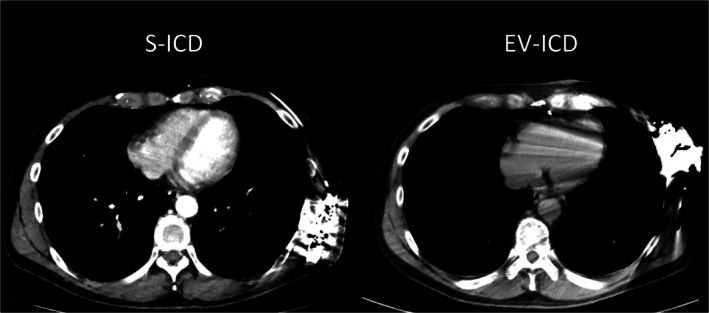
Pre‐ and postconversion computed tomography demonstrating that the final extravascular implantable cardioverter‐defibrillator generator position was immediately anterior to and closely aligned with the original subcutaneous implantable cardioverter‐defibrillator generator site.
